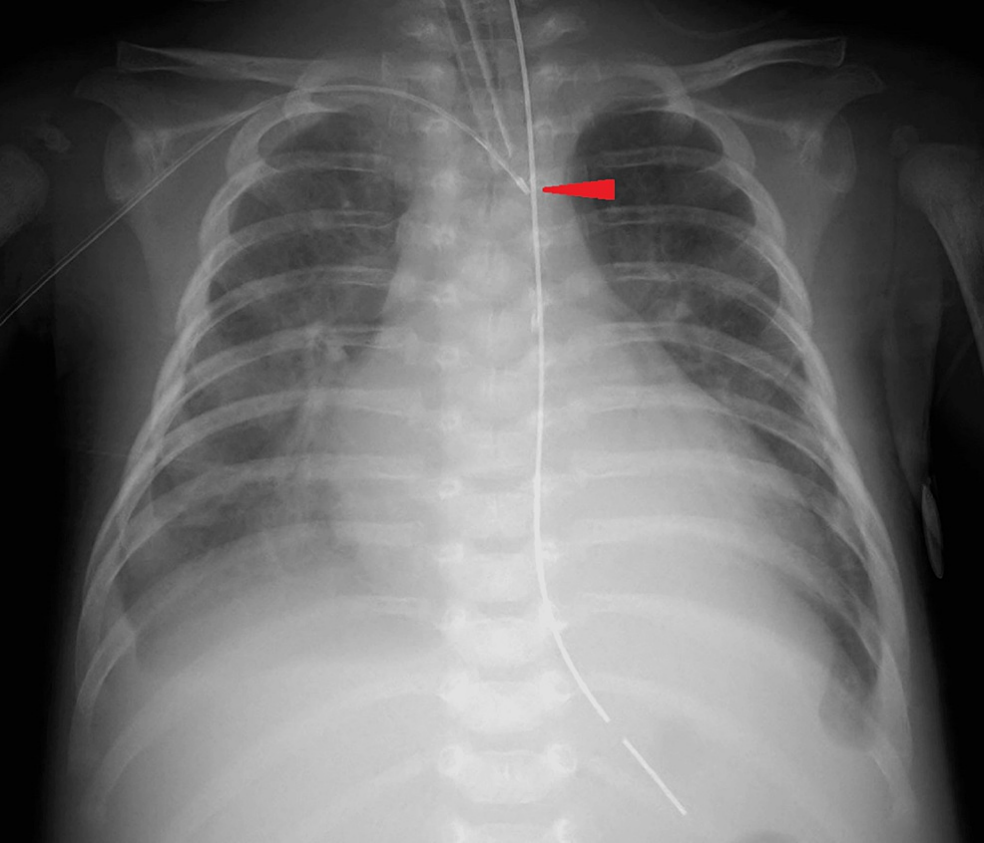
Chest radiography after insertion of a peripherally inserted central catheter The arrowhead shows the tip of the peripherally inserted central catheter at the aorta.
